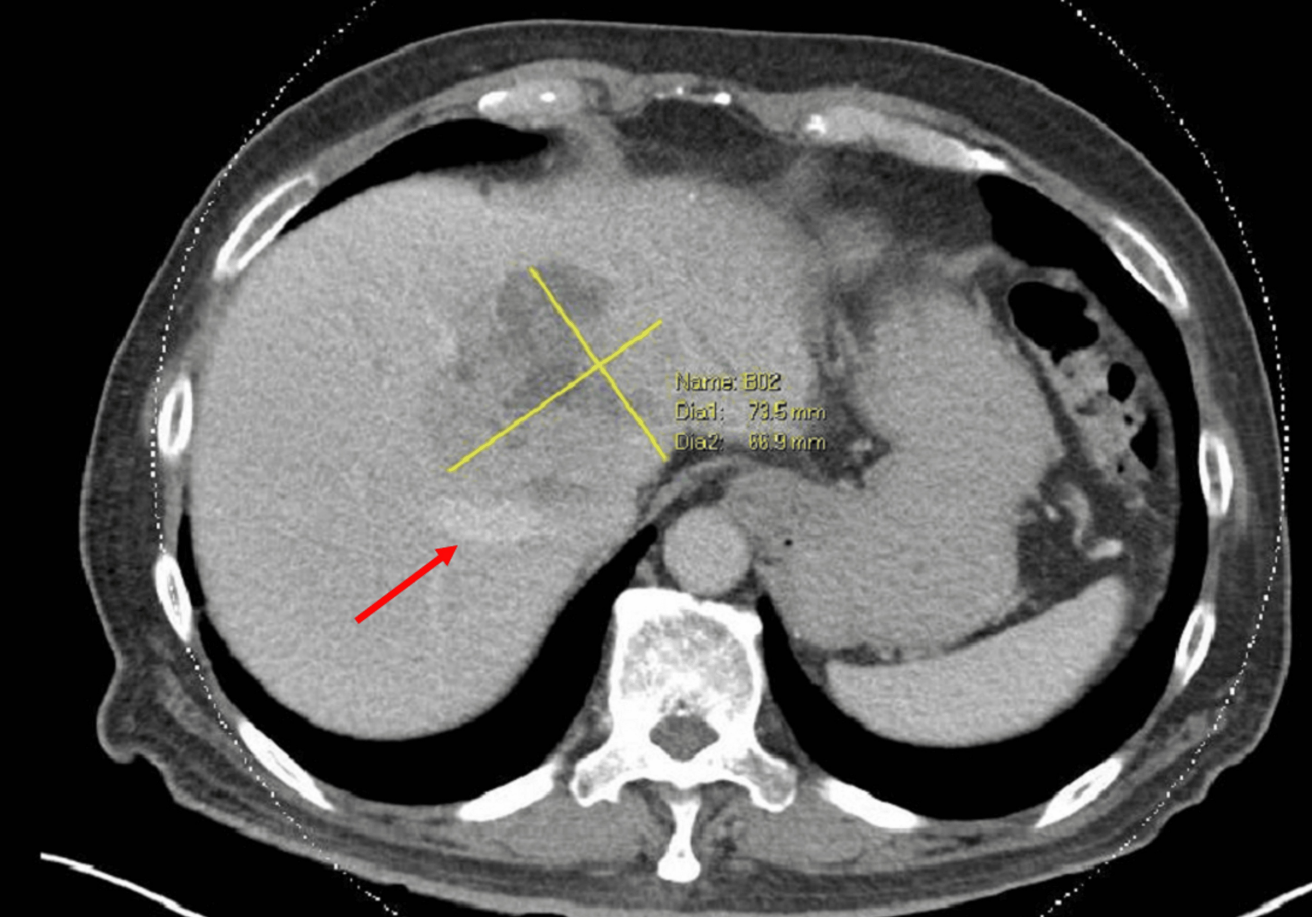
Axial CT venous phase again demonstrating a large, centrally located lesion abutting the middle hepatic vein (red arrow).
